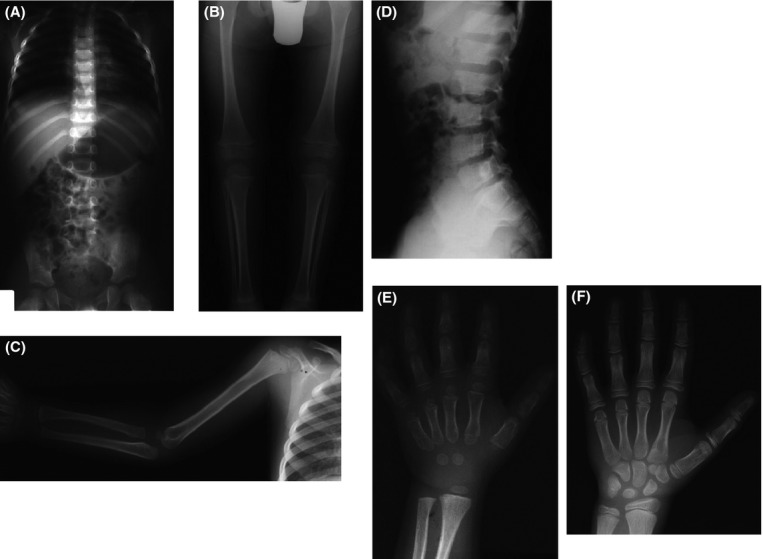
Radiographs of a case of hypochondroplasia with a p.Gly380Arg mutation (R# 92-231A). (A) AP chest and pelvis, age 5 years. The sacrosciatic notch is narrowed, but there is no interpediculate narrowing. (B) AP legs, age 5 years. There is distal fibular overgrowth. (C) AP arm, age 5 years. There is only mild rhizomelic shortening. (D) Lateral lumbar spine, age 12.5 years. There is mild platyspondyly and a narrowed spinal canal. (E) PA left hand, age 5 years. Note the brachydactyly and brachymetacarpia. (F) PA, left hand, age 12.5 years. The brachymetacarpia is less pronounced than at age 5.
